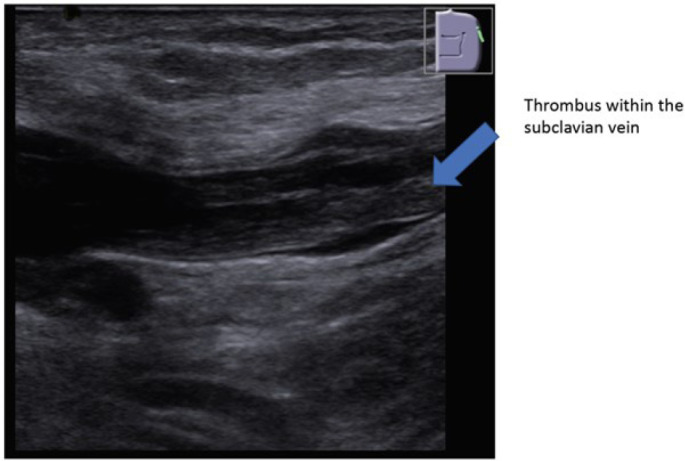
Duplex sonography showing thrombosis of the left subclavian vein.
